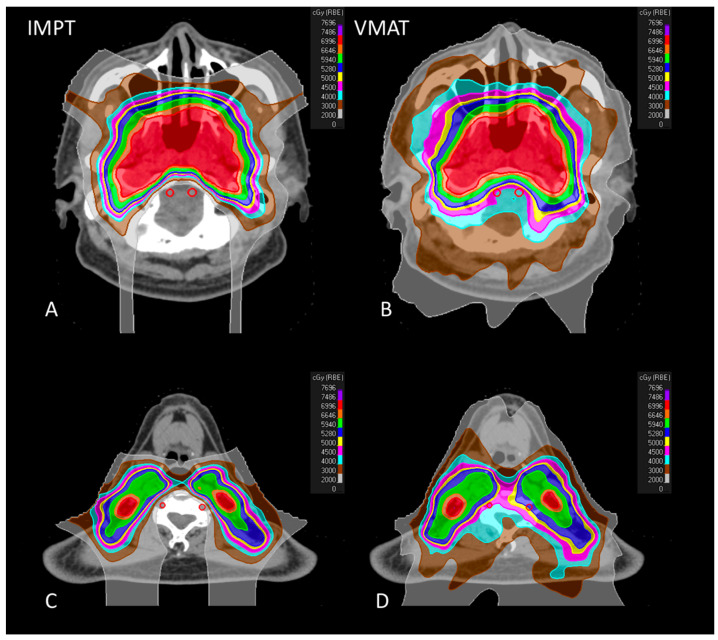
Representative axial CT images of colorwash dose distribution comparison for IMPT (left side) and VMAT (right side) plans of the same patient. (A): the dose distribution located at skull base level IMPT; (B): the dose distribution located at skull base level VMAT; (C): the dose distribution at the level of the fourth cervical vertebra of IMPT; (D): the dose distribution at the level of the fourth cervical vertebra of VMAT.
